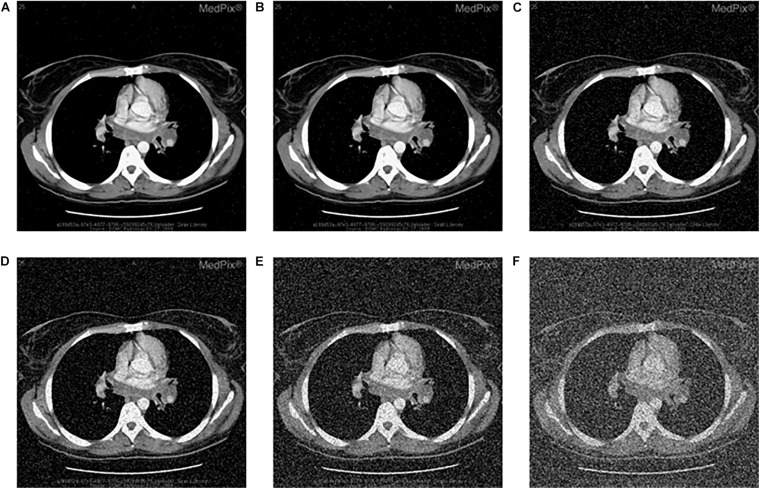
Salt-and-pepper noise attack of encrypted CT-chest, decrypted image with density value (A) 0.002, (B) 0.005, (C) 0.05, (D) 0.1, (E) 0.25, and (F) 0.5.
